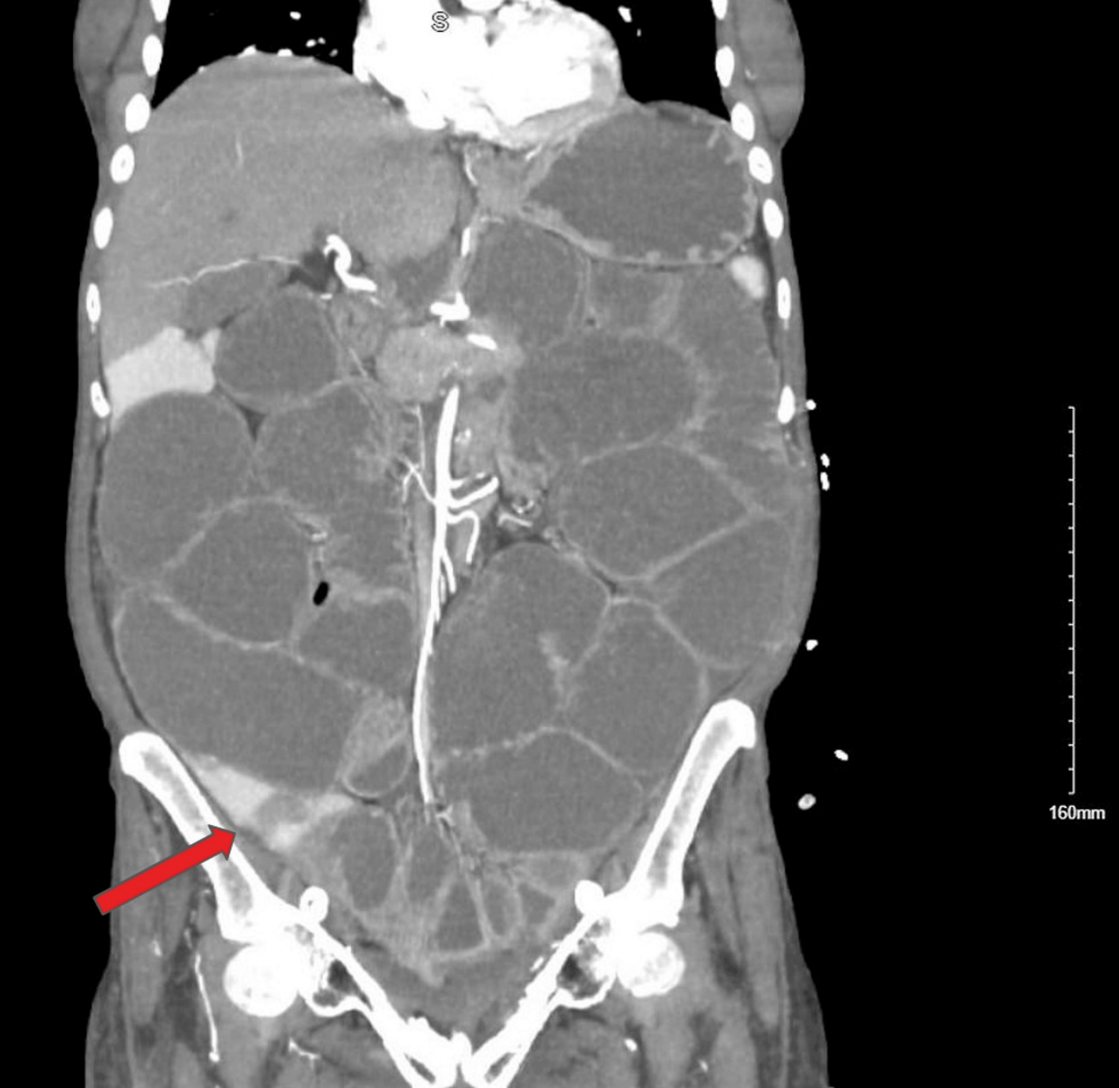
Computed tomography angiography of the abdomen and pelvis demonstrating dilated loops of small bowel with a transition point in the pelvis (arrow), consistent with mechanical small bowel obstruction. The transition point represents the site of obstruction where the proximal bowel is dilated and the distal bowel is decompressed.
